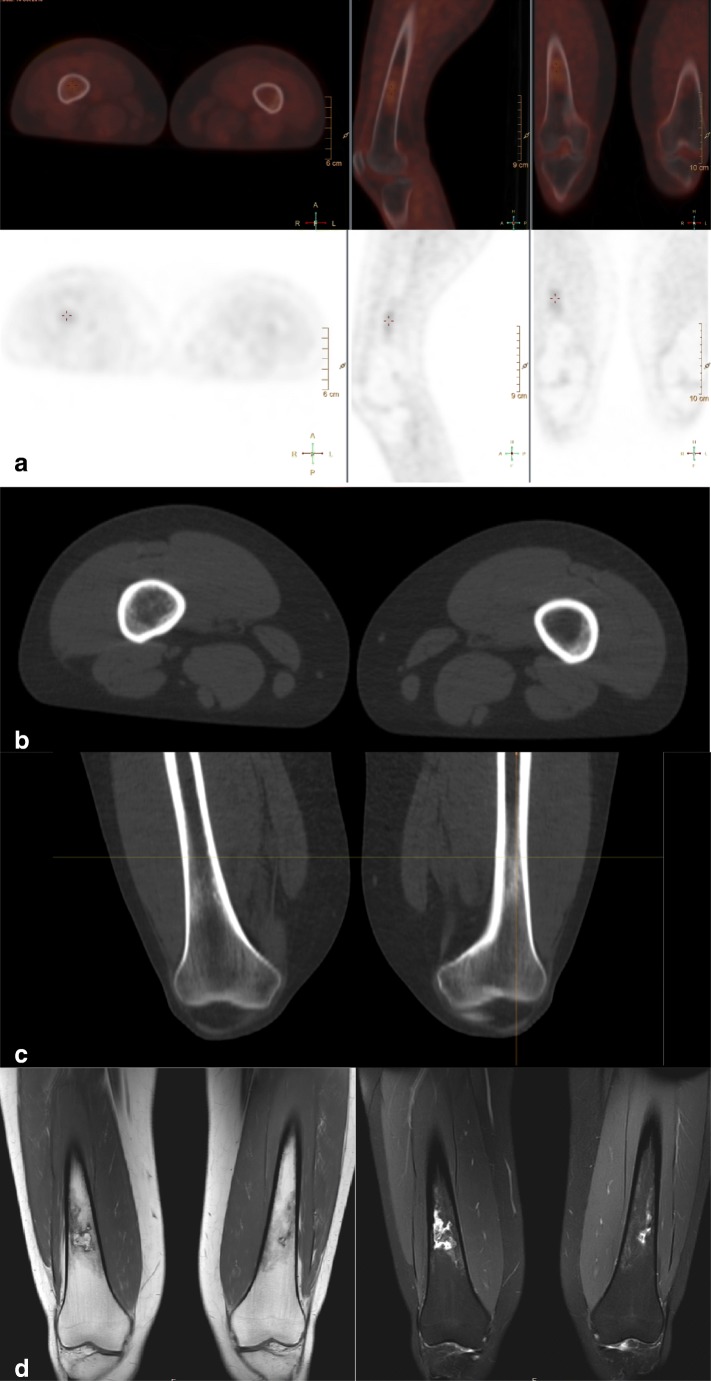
False-positive lesions on ^18^F-FDG PET-CT. A 19-year-old woman diagnosed with localized Ewing sarcoma of the seventh rib. Six months after initial treatment consisting of six courses of vincristine, ifosfamide, doxorubicin, and etoposide (VIDE) chemotherapy, 8 courses of vincristine, actinomycin-D, and ifosfamide (VAI) chemotherapy, radiation therapy and surgery, imaging was performed because of chest pain, with local recurrence suspected. a^18^F-FDG PET-CT showed two lesions with ^18^FDG-uptake in both femora of which the true nature could not be clearly defined; based on the information available they were classified as inconclusive (positive). b and c Low-dose CT images in the transverse and coronal planes of the suspected bone lesions showing sclerosis. d MRI T1- and T2-weighted images show bilateral bone infarctions and no sign of malignancy
